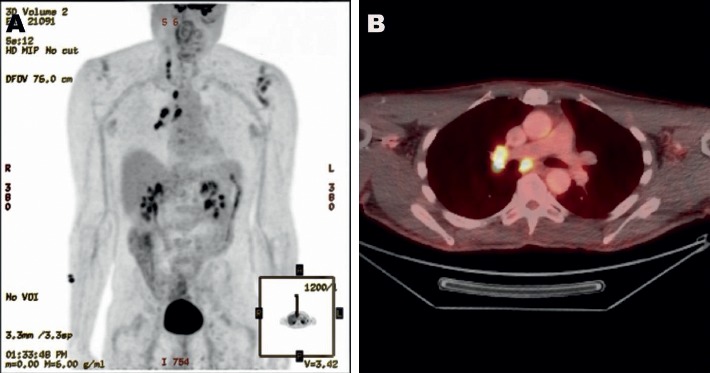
(a) Contrast-enhanced FDG-PET showing hypermetabolic lymph nodes in the right upper and lower paratracheal nodes, right hilar nodes, and subcarinal nodes. (b) Hypermetabolic subcarinal and right hilar lymph nodes.
